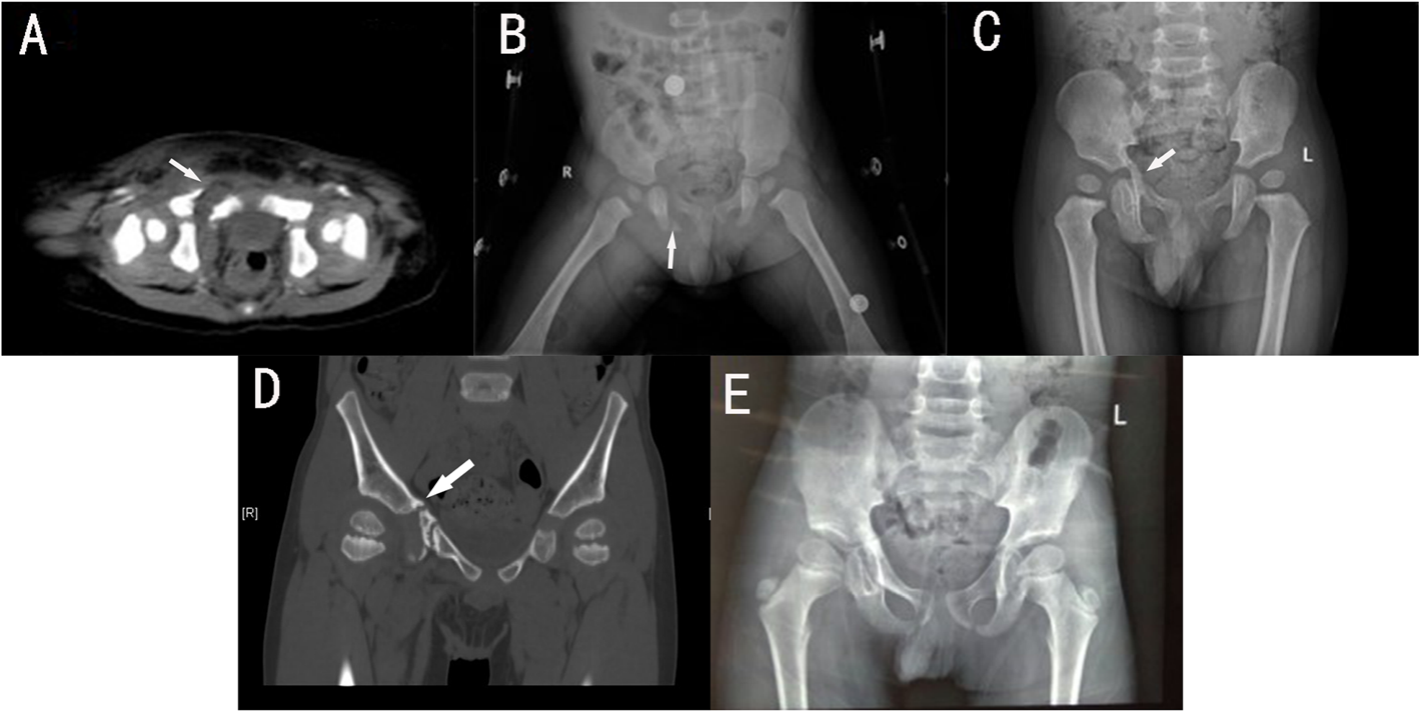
A 1.4-year-old boy (case C). X-ray and CT suggests fractures of superior and inferior ramus of pubis and right ischium (white arrow) (A, B). Two weeks later, X-ray showed a faint bony bridge formation across the medial margin of the triradiate cartilage on the right acetabulum (C) (white arrow). Five months after the injury, X-ray showed rupture of the original bone bridge (white arrow) (D). Seven years after injury, X-ray showed that the triradiate cartilage remained open, but the medial wall of the right acetabulum had thickened, the acetabulum had become shallow, and the femoral head had shifted slightly laterally (E)
